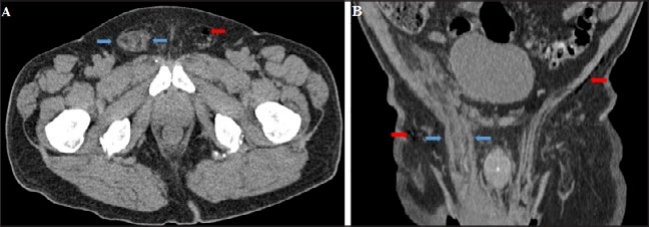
Unenhanced CT-scan of the pelvis, axial source image (A) and coronal reformatted image (B) shows the inflamed right spermatic cord, which cause distension of the inguinal canal (arrows blue). Subcutaneous emphysema and gas bubles within the left inguinal canal also were present (arrows red).
